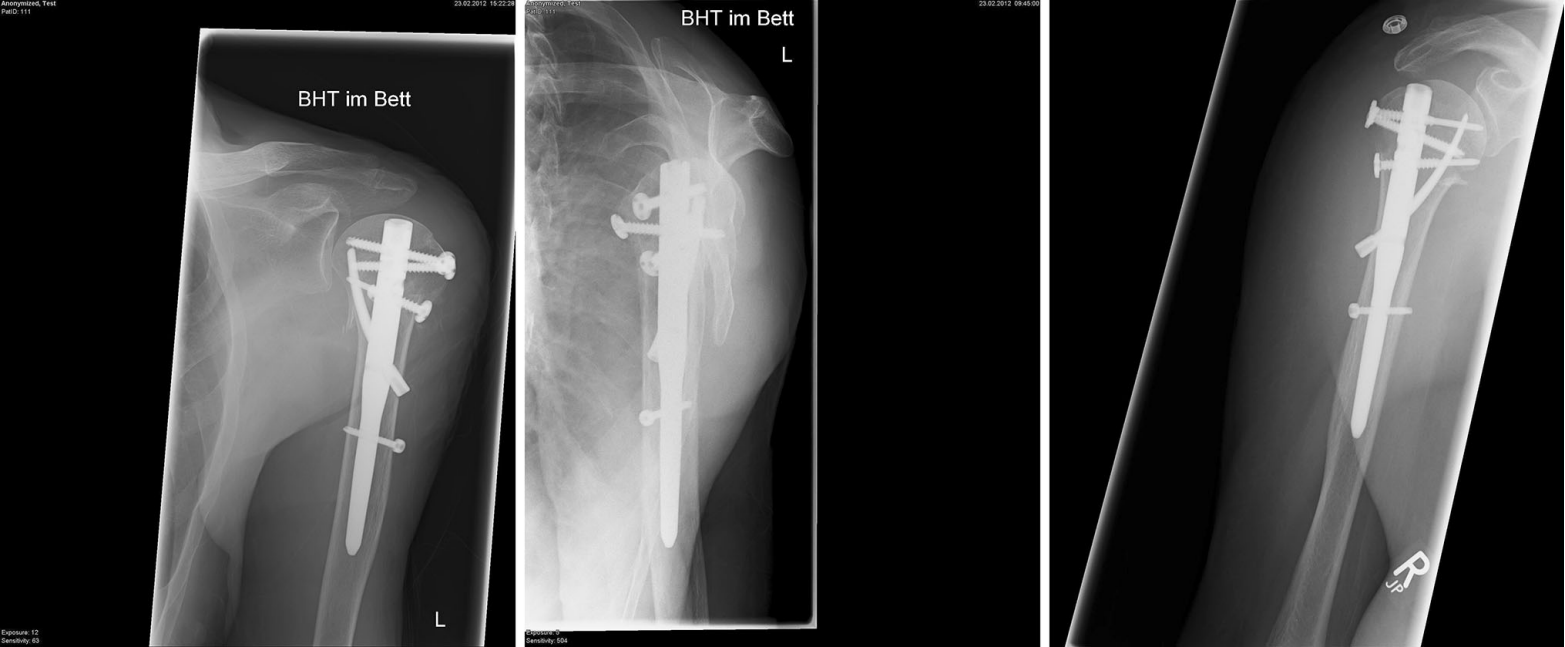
Post-operative radiograph showing LBN in position
